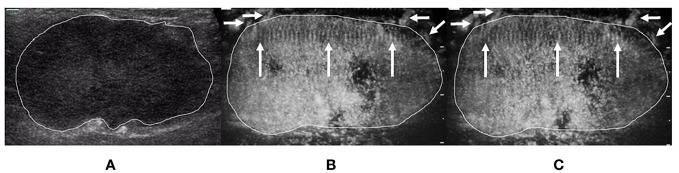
A 75-year-old woman with invasive breast carcinoma proven by pathology. The tumor region is marked in white. (A) Grayscale ultrasound image of breast tumor. (B) The ≤30-s phase: the tumor increased in scope compared with the grayscale ultrasound. Microvasculature morphology shows a crab claw-like pattern (short arrows), with multiple parallel small spiculated vessels (long arrows) in the peripheral region of the tumor. (C) The >30-s phase: the tumor increased in scope compared with the grayscale ultrasound. Microvasculature morphology shows a crab claw-like pattern (short arrows), with multiple parallel small spiculated vessels (long arrows) in the peripheral region of the tumor.
